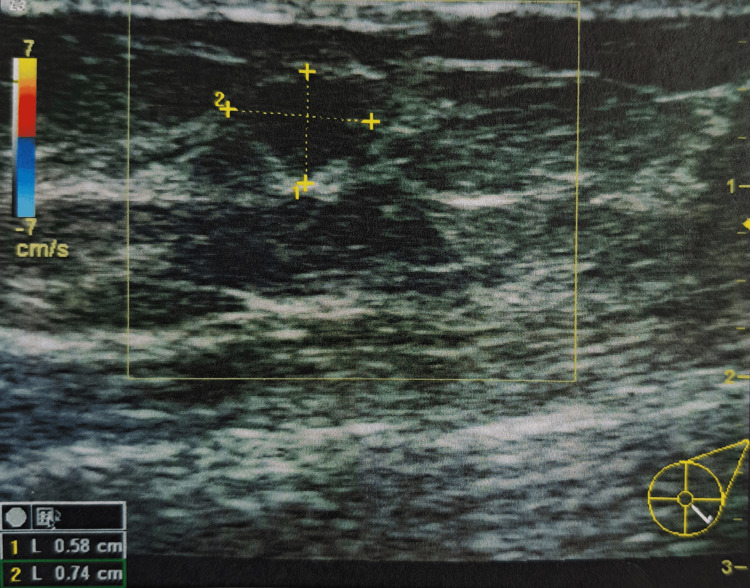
Breast ultrasound showing a hypoechoic nodule 0.6 x 0.53 x 0.74 cm with lobulated borders, at the four o'clock position of the left breast
